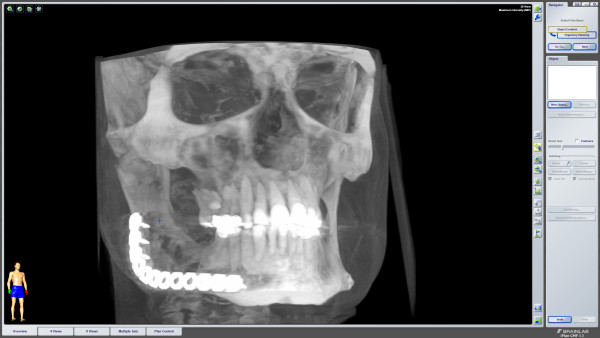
Postoperative CBCT showing the defect and the titanium plate.
